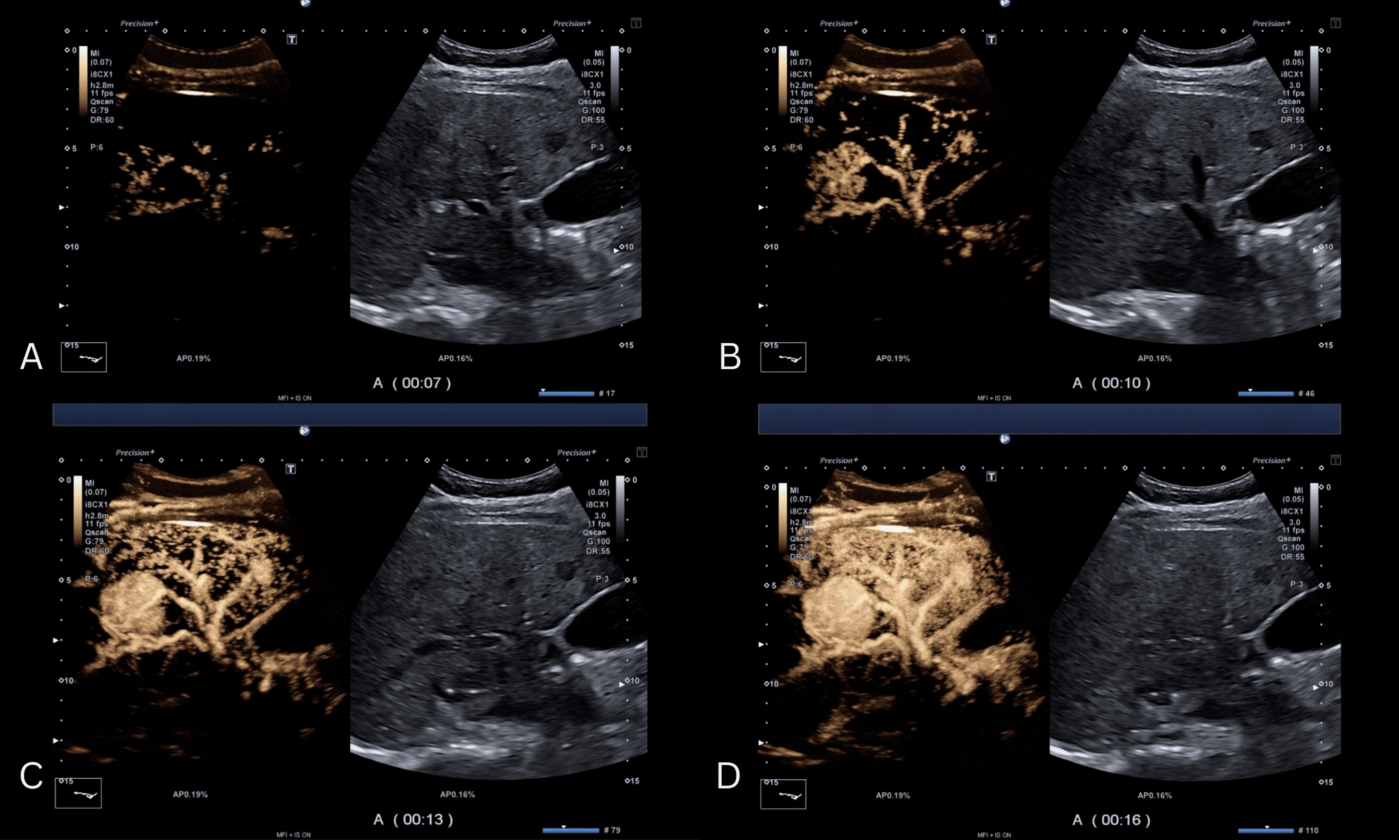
Sequential CEUS imaging demonstrating centrifugal contrast filling of branching arteries. (A–D) Time-lapse series showing progressive enhancement CEUS: contrast-enhanced ultrasound
